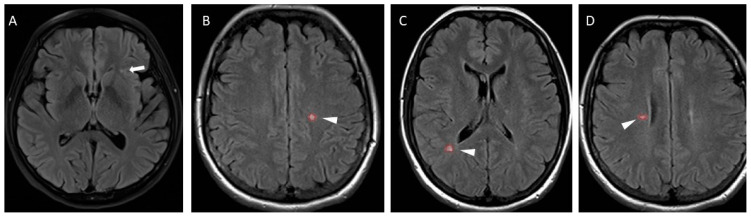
MRI images of multiple sclerosis (MS) and nonspecific white matter lesion (NSWMLs) patients. (A) Axial fluid-attenuated inversion recovery (FLAIR) images of NSWMLs patients showing a subtle white matter lesion located in deep white matter (white arrow). (B–D) Axial FLAIR images with hyperintense lesions in typical locations for MS (arrowhead).
